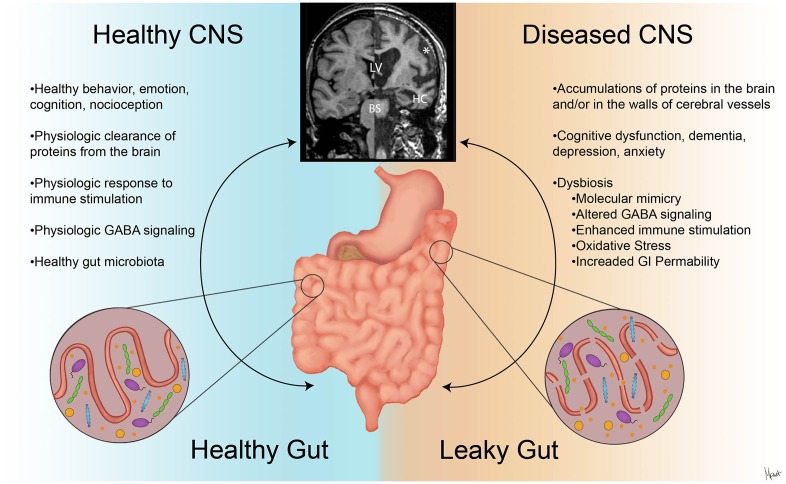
The contrasts of clinical presentations on the GBA in health and neurodegenerative diseases. A stable intestinal microbiota is essential for healthy gut physiology and contributes to appropriate signaling along the GBA, promoting healthy physiologic status as well as central nervous system (CNS) status (*left*). Intestinal dysbiosis can negatively influence gut physiology and lead to abnormal GBA signaling (Friedland, 2015), resulting in accumulation of misfolded amyloid species (Galloway et al., 2007; de Lartigue et al., 2011). This can ultimately alter CNS functions and anatomy (Wu et al., 2017) as shown with magnetic resonance imaging (MRI) volumetric scans (*upper middle*). In the diseased CNS and gut state (*right*), cortical atrophy with the widening of the subarachnoid space (*), enlargement of the lateral ventricles (LV), hippocampus atrophy (HC), and brainstem (BS) volume reduction are seen with clinical and cognitive dysfunction (Johnson et al., 2012; Lee et al., 2015).
